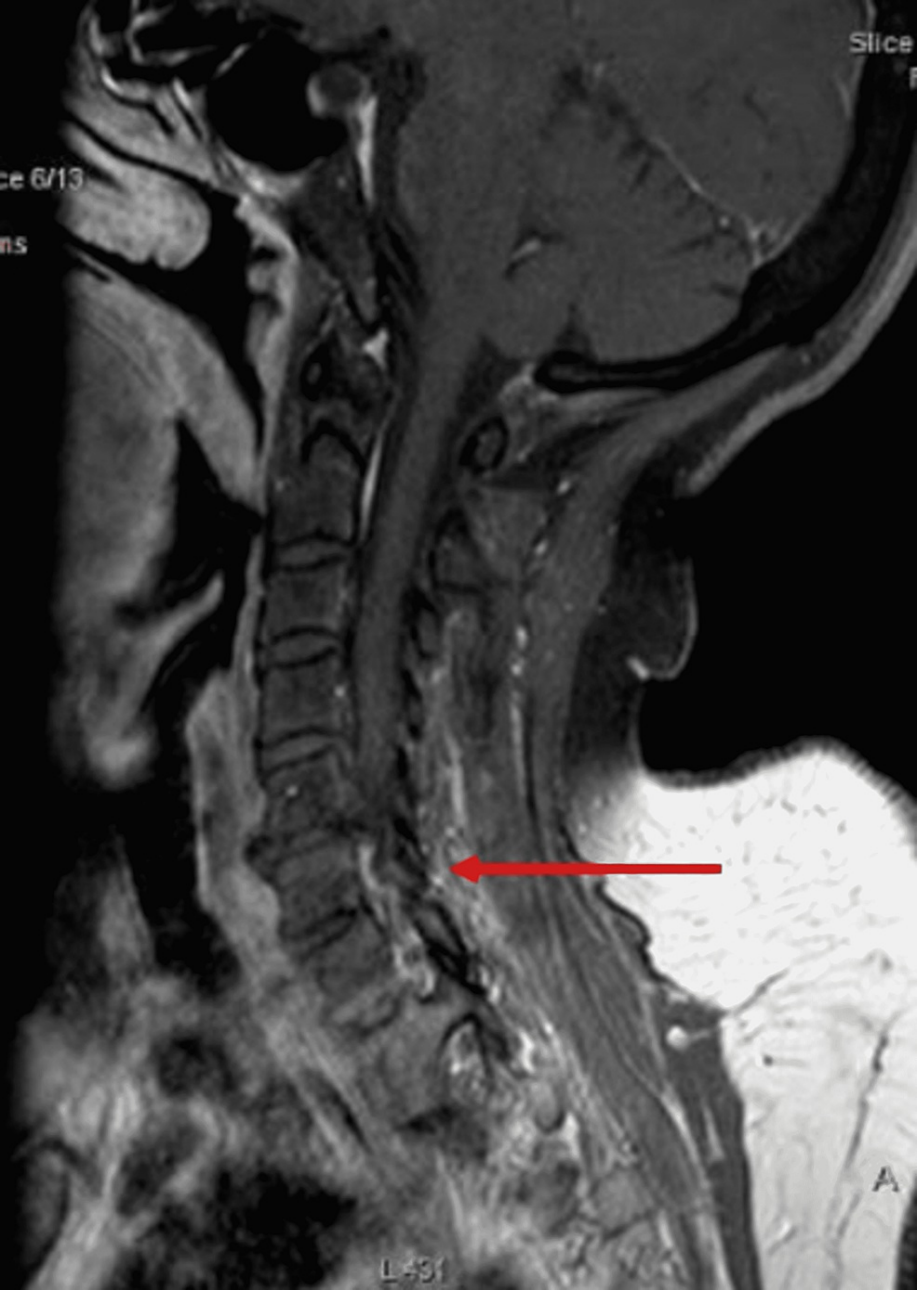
MRI of the cervical spine showing severe canal stenosis at C4-C5, C5-C6, and C6-C7, and multilevel severe neural foramina stenosis (red arrow).
